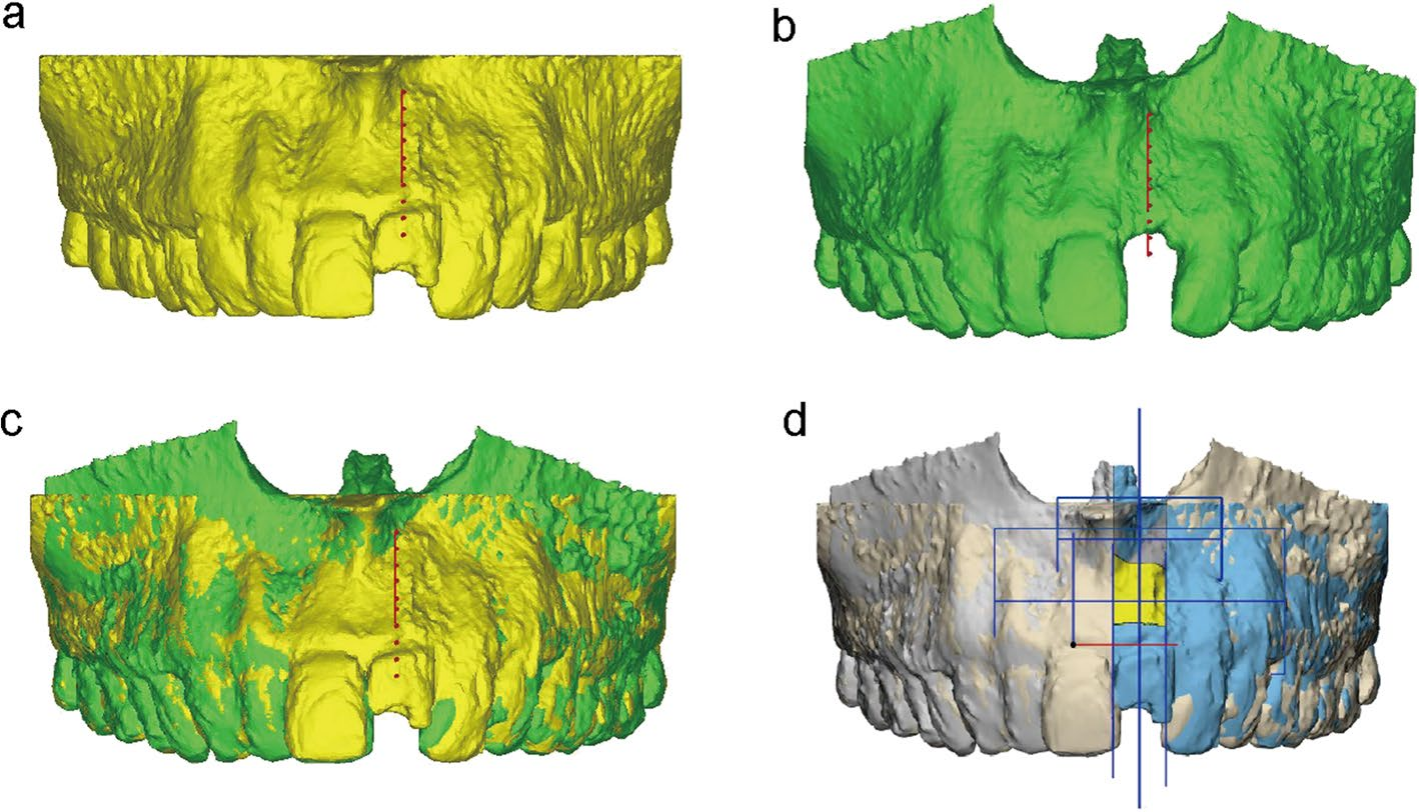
a 3D virtual reconstruction of CBCT data at T0. b 3D virtual reconstruction of CBCT data at T1. c Superimposition of virtual models at T0 and T1. d ROI (yellow) in the superimposed model
